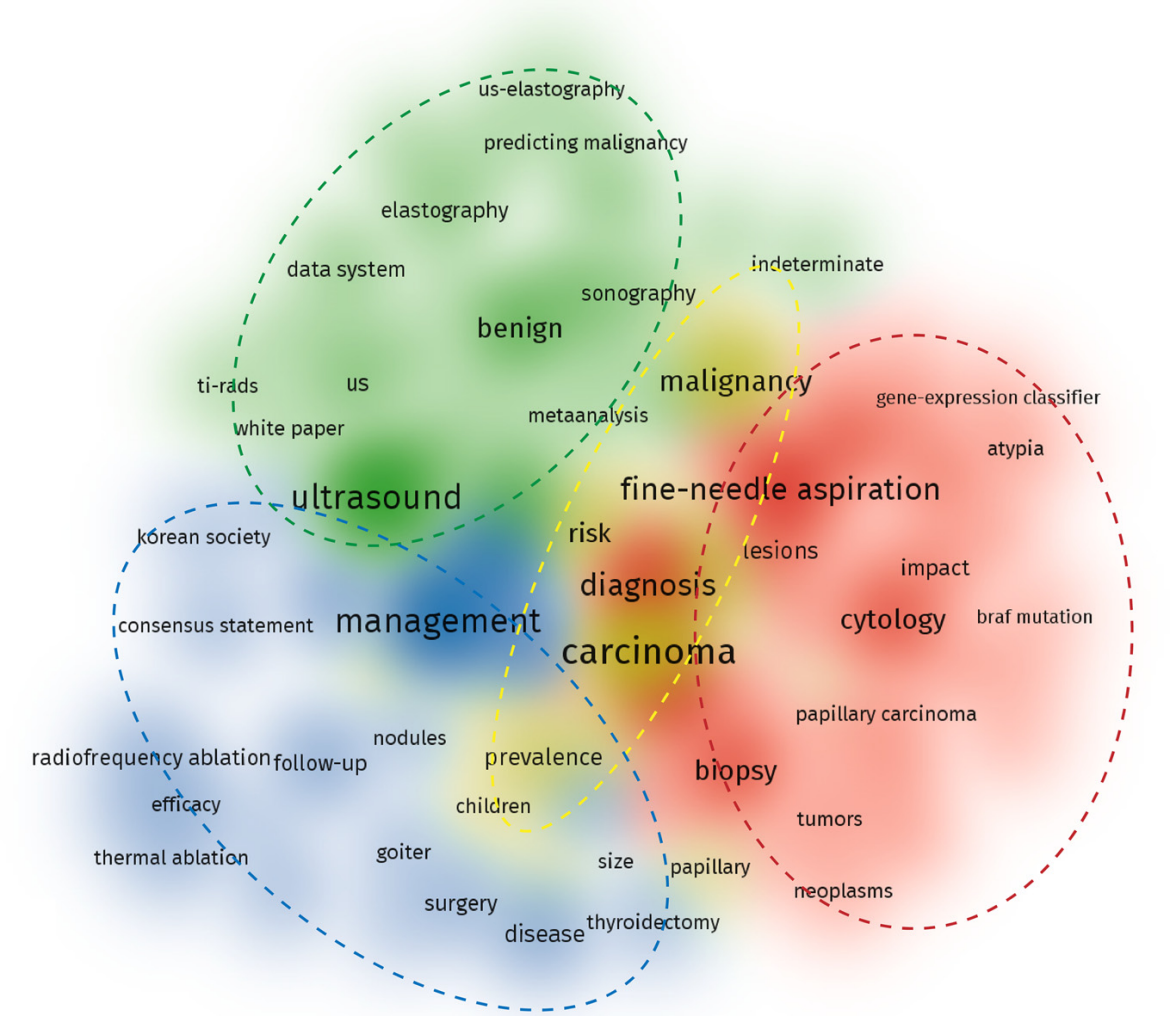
Topic clusters sorted by VOSviewer: Red, fine-needle aspiration and cytology in the diagnosis of TNs; green, ultrasound and benign features of TNs; yellow, thyroid carcinoma and malignancy of TNs; and blue, treatment and management of TNs. The gradation of circle colour represents the number of publications for each topic.
